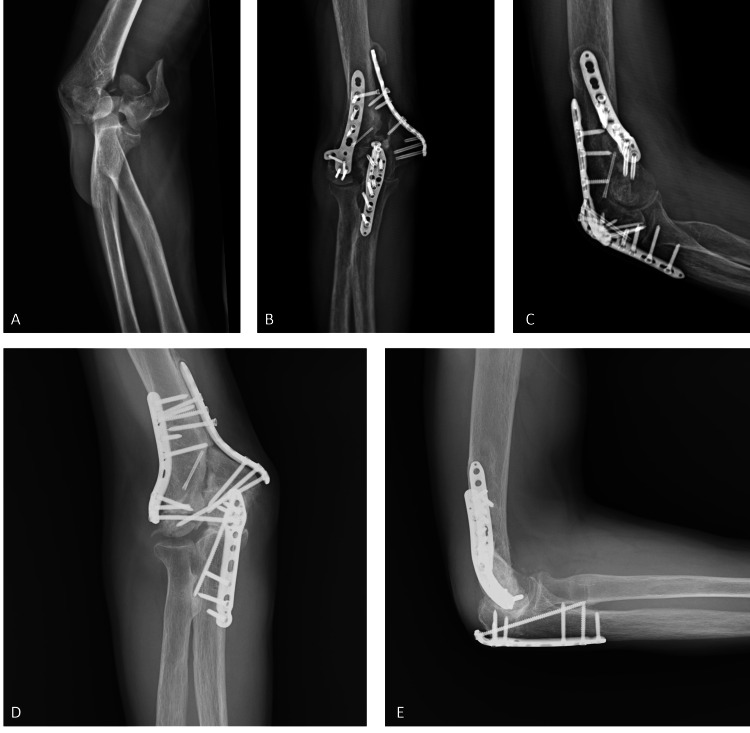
Radiographs of the patient after trauma, first surgery, and revision surgery. (A) Anteroposterior elbow radiograph of the patient after the trauma showing the bi-columnar distal humerus fracture. (B) Anteroposterior elbow radiograph of the patient before the revision surgery showing distal humerus nonunion and implant failure. (C) Lateral elbow radiograph of the patient before the revision surgery showing distal humerus nonunion and implant failure. (D) Anteroposterior elbow radiograph of the patient after the revision surgery. (E) Lateral elbow radiograph of the patient after the revision surgery.
